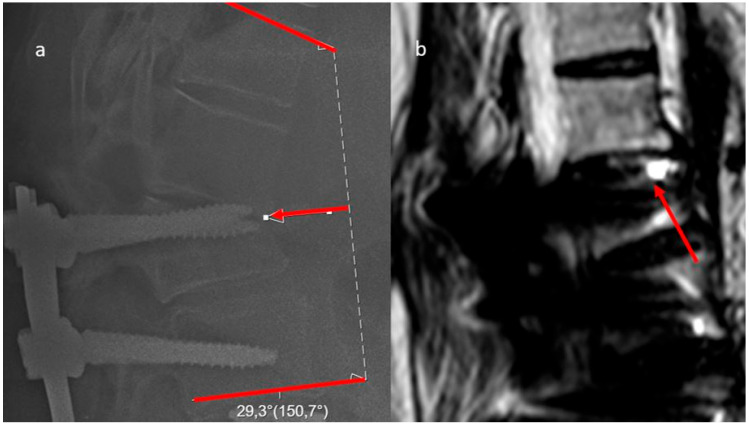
Proximal clinical adjacent segment disease with junctional failure in a 66-year-old woman. (a) Lateral radiograph demonstrating proximal junctional kyphosis above the instrumented construct, accompanied by failure of the cranial endplate of the upper instrumented vertebra. The angular marker and arrows indicate the kyphotic transition zone and structural failure at the proximal junction. (b) Sagittal magnetic resonance image showing adjacent disc disruption at the proximal junctional level. This figure demonstrates the transition from proximal radiographic junctional change to a clinically relevant ASD phenotype, where kyphotic collapse, endplate failure, and adjacent disc disruption together represent mechanical decompensation of the transition zone.
